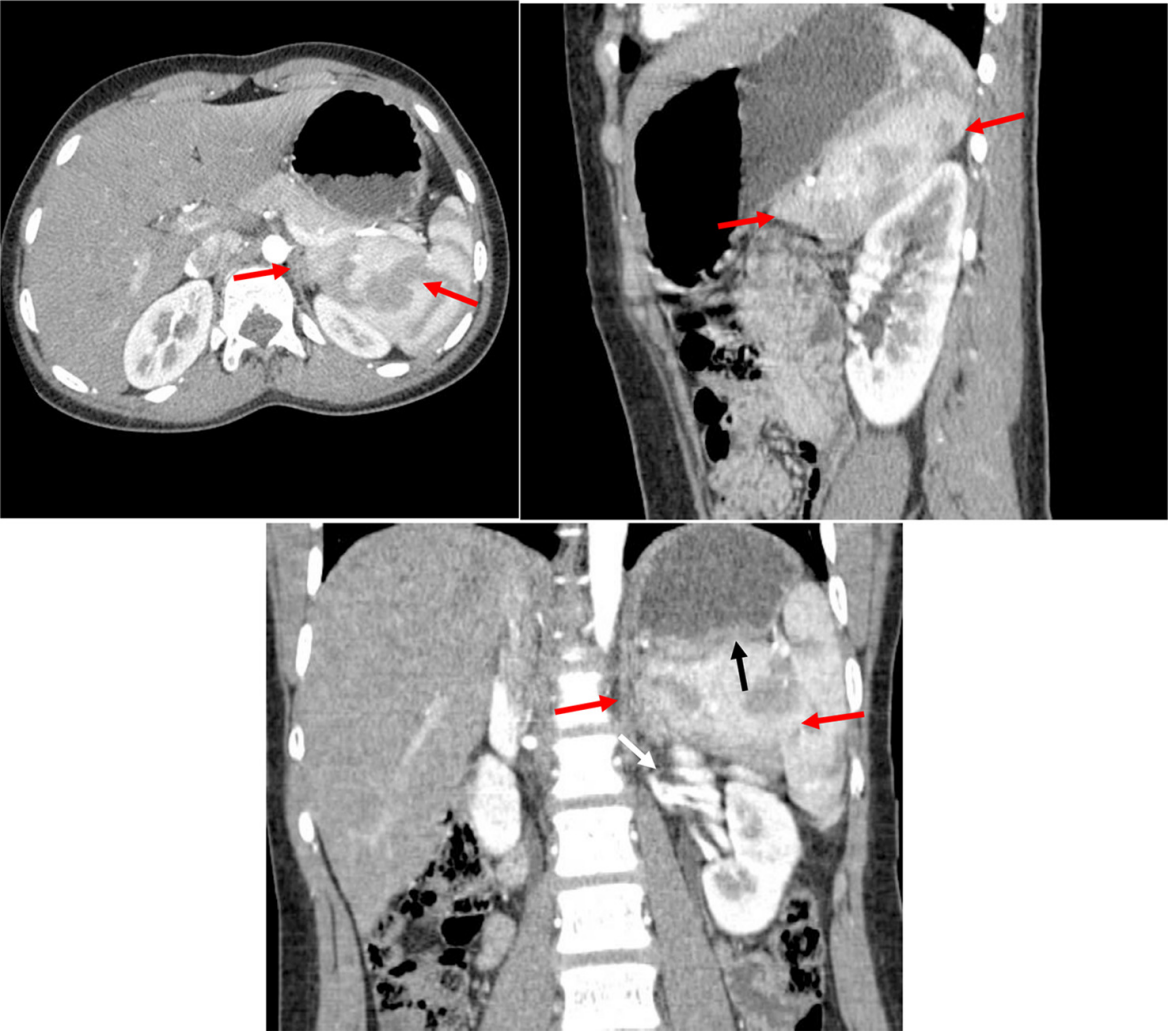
Concentric axial, sagittal and coronal multiplanar reformations contrast-enhanced CT images acquired during the arterial phase through the mid-spleen demonstrates the medial (internal) lobe of the spleen (red arrows) shows homogeneous CT density and isodense to the reminder of the spleen. The medial (internal) lobe of the spleen extends medially displacing the upper pole of the left kidney (white arrow) downwards and gently indenting the posterior aspect of the gastric fundus (black arrow). Both the medial (internal) and lateral (external) splenic lobes show mottled (zebra lines) pattern.
